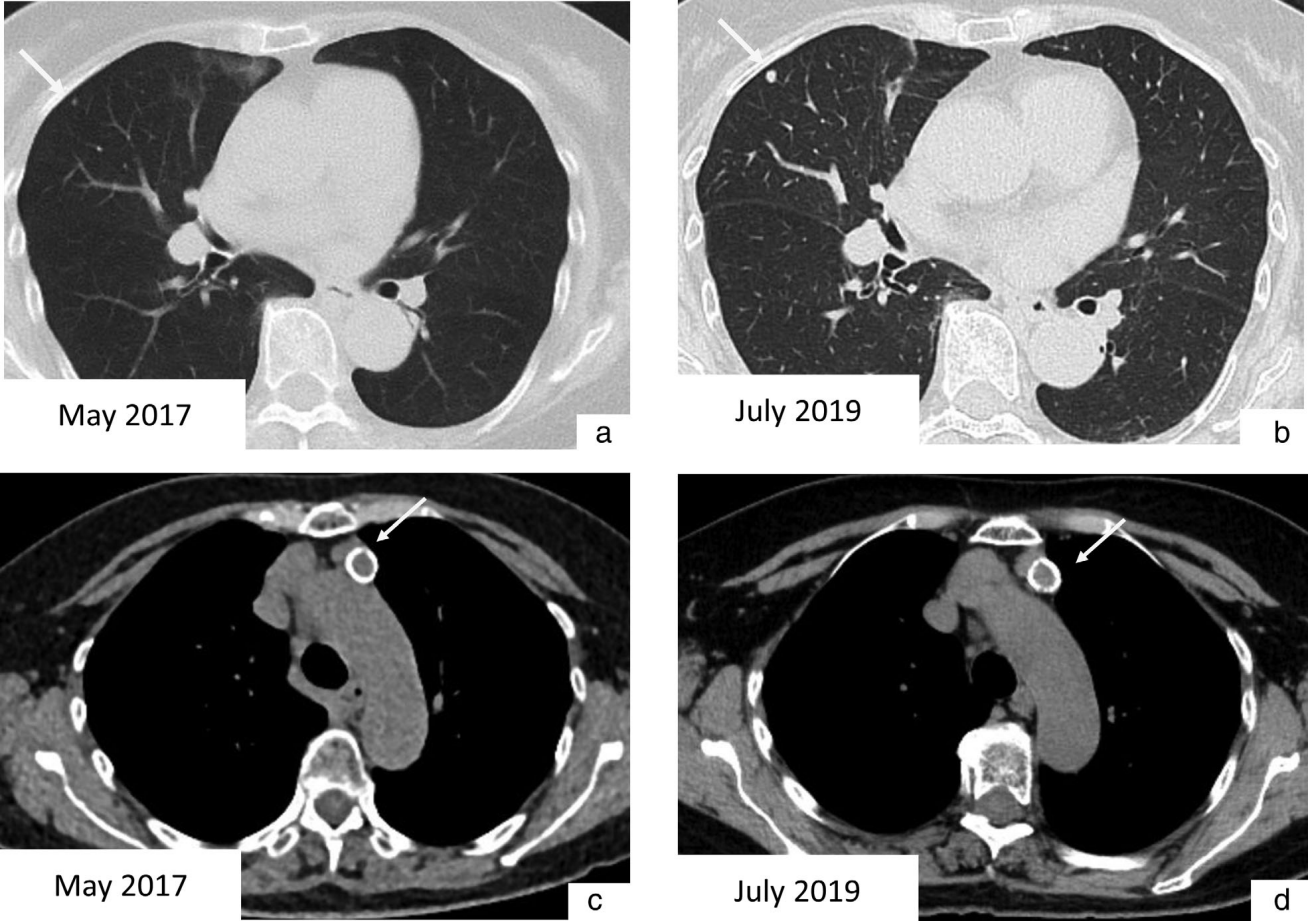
(a) A pulmonary nodule in the right middle lobe was detected by chest computed tomography (CT). (b) It had grown slightly over a two‐year period. (c) An anterior mediastinal tumor with ring‐shaped calcification was detected by chest CT. (d) It had not changed markedly over a two‐year period
